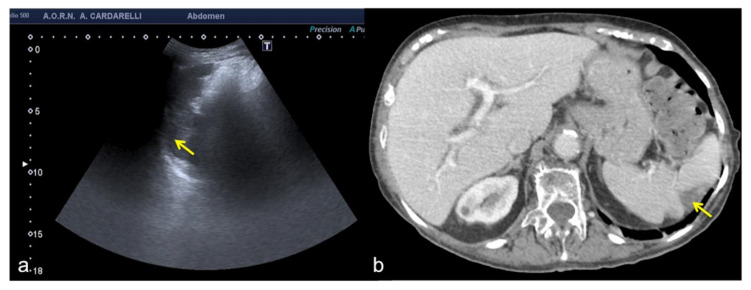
Coronal US scan of the left hypochondrium (a) shows partial exploration of the spleen with unrecognized traumatic injury (arrow) in a 22-year-old man investigated for trauma. On CT scan (b) it appears to be more evident (arrow).
